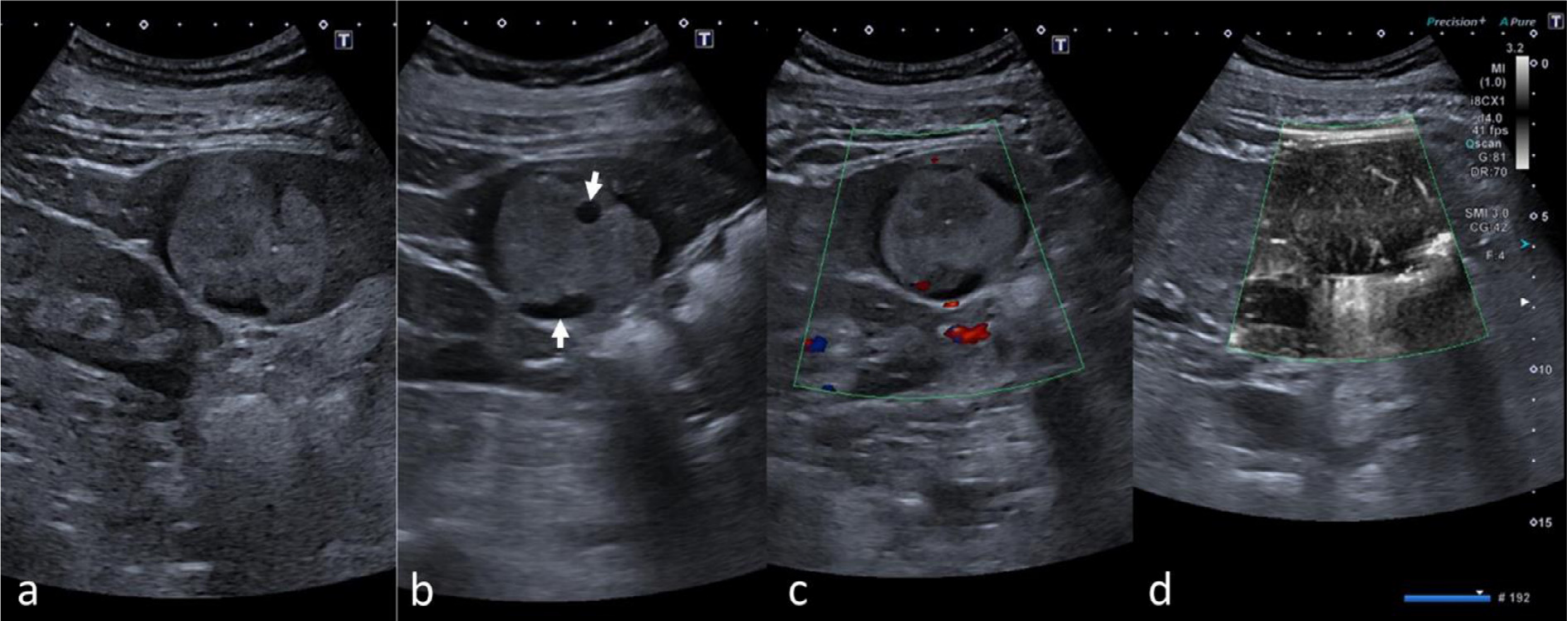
B-Mode and Doppler imaging. Peliosis hepatis demonstrating as a hyperechogenic solitary lesion (A) with hypoechogenic halo and cystic compartiments (B, arrows). On color-coded Doppler sonography, no macrovascularization was noted (C), but microvascular imaging showed small, low-flow vessels inside the lesion.
